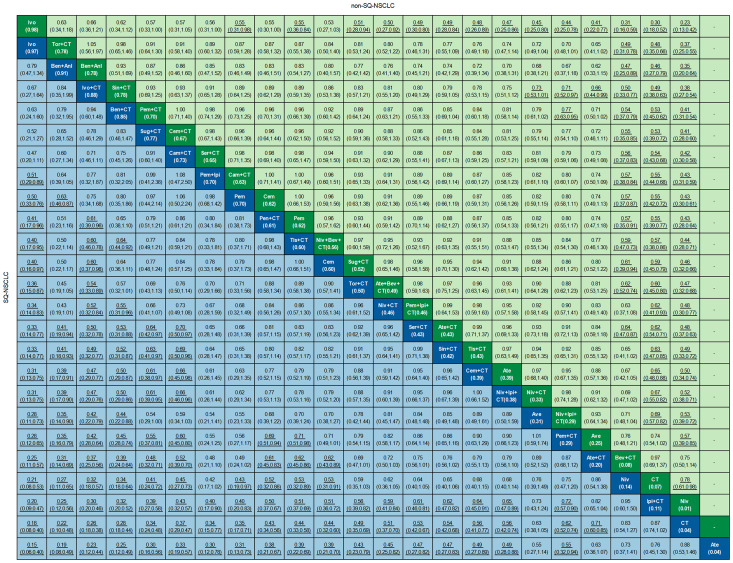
Pooled PFS estimates of multiple comparisons in network meta-analysis. Treatment regimens are presented in order of PFS ranking from high to low according to SUCRAs. Dark blue represents the regimens for SQ-NSCLC (SUCRA values), and dark green represents the regimens for non-SQ-NSCLC (SUCRA values). Data are HRs (95% CrIs) for column-defined treatment versus row-defined treatment for SQ-NSCLC (lower triangle) and row-defined treatment versus column-defined treatment for non-SQ-NSCLC (upper triangle). Significant results are underlined.PFS, progression-free survival; SUCRA, surface under the cumulative ranking; HR, hazard ratio; CI, confidence interval; SQ-NSCLC, squamous non-small cell lung cancer; Anl, anlotinib; Ate, atezolizumab; Ave, avelumab; Ben, benmelstobart; Bev, bevacizumab; Cam, camrelizumab; Cem, cemiplimab; Dur, durvalumab; Ipi, ipilimumab; Ivo, ivonescimab; Niv, nivolumab; Pem, pembrolizumab; Pen, penpulimab; Ser, serplulimab; Sin, sintilimab; Sug, sugemalimab; Tis, tislelizumab; Tor, toripalimab; Tre, tremelimumab; CT, chemotherapy.
